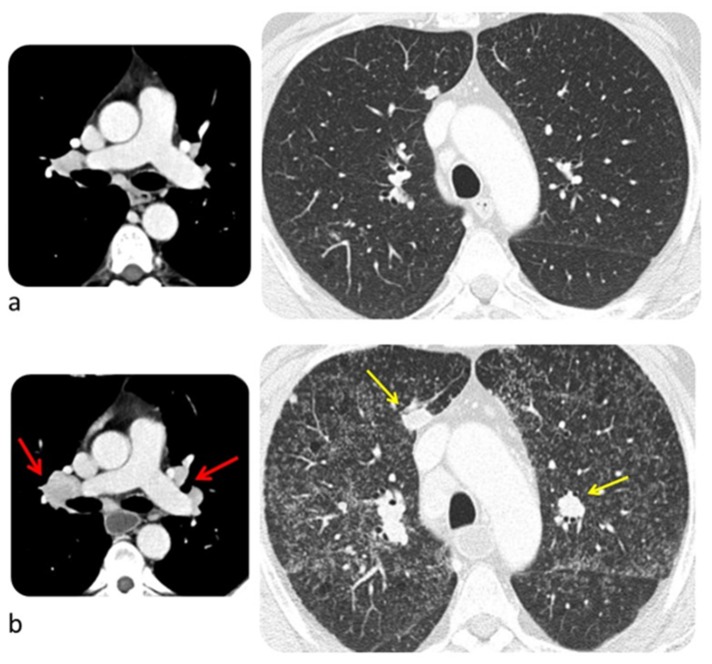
Sarcoid-like post immunotherapy granulomatosis in a 48-year-old man with metastatic melanoma. CT scan was performed before starting pembrolizumab (a) and two months later (b). The latter shows appearance of disseminated small nodules similar in size, enlarged hilar and mediastinal lymph nodes (red arrow), and progressive disease (yellow arrow). © Department of Radiology, Candiolo Cancer Institute—IRCCS. Turin.
